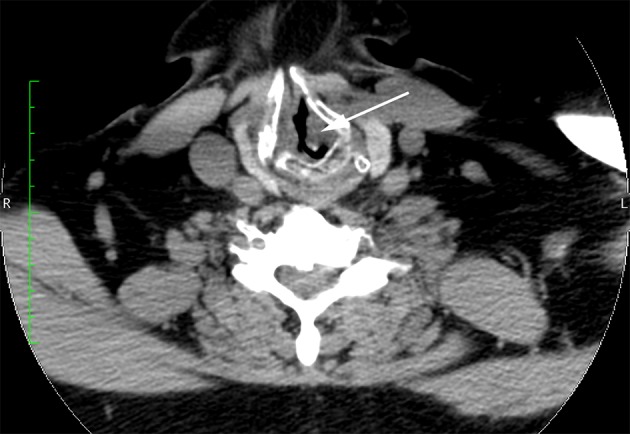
Enlarged Axial Computed Tomographic Scan Demonstrating Subglottic Stenosis (Arrow) and Narrowed Airway Lumen
